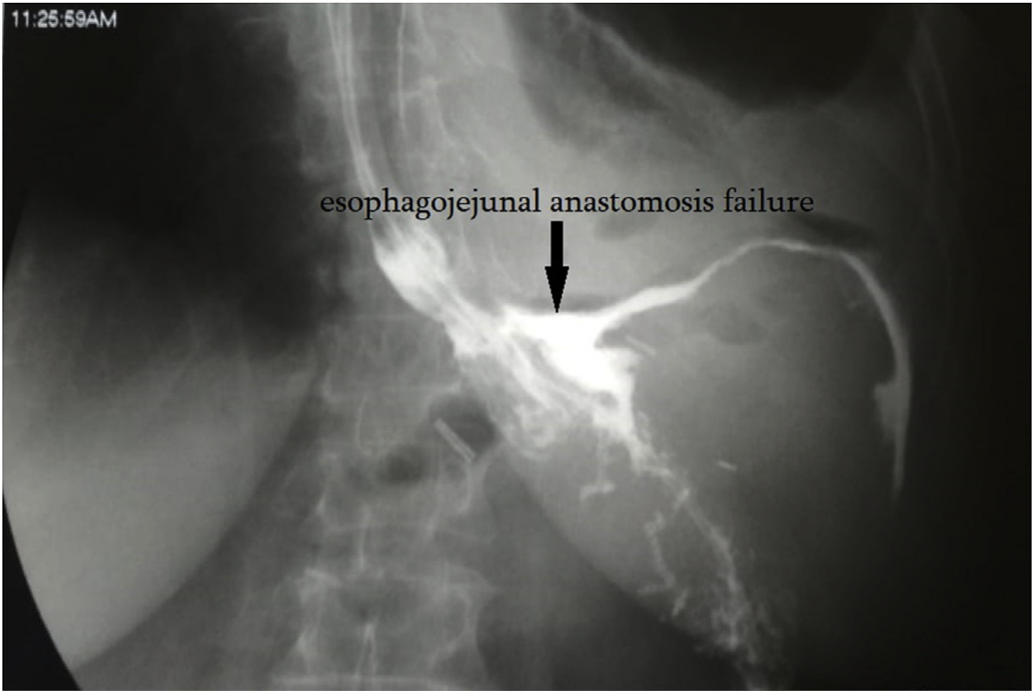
Contrast X-ray: failure of esophagojejunal anastomosis with the formation of fistula.
